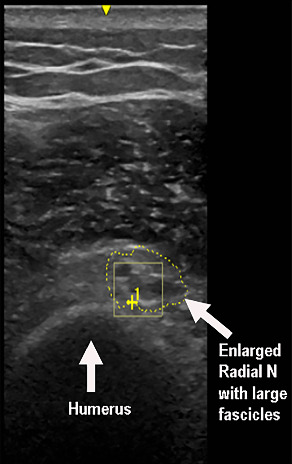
Ultrasound showed enlargement of the radial nerve with large fascicles at the spiral groove.
